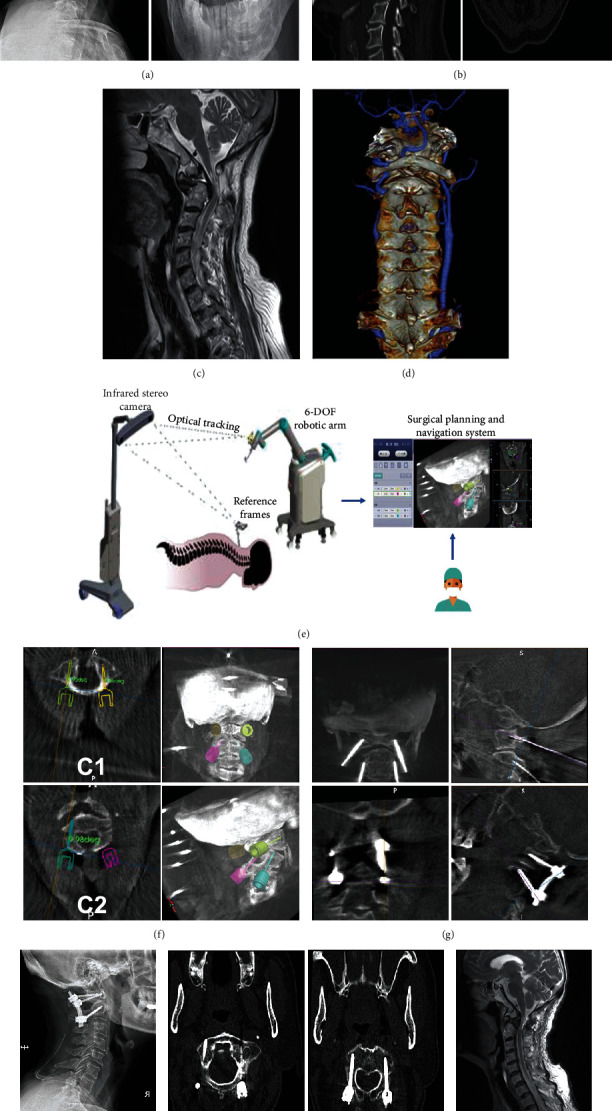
Case presentation. A 65-year-old female patient presented with a 3-year-long history of progressive dizzy, numbness, and weakness of extremities. (a, b) X-ray and CT examinations indicated os odontoideum and atlantoaxial instability (white arrows). (c) Preoperative magnetic resonance imaging (MRI) indicated a high signal intensity in the medulla oblongata. (d) No changes in VA course were observed in the 3D-CTA images. (e) The working principles of the TiRobot system. (f) Surgical planning of trajectories in the TiRobot system. (g) Robot-assisted insertion of C1LMS and C2PS. (h–j) X-ray, CT, and MRI images at 3 months postsurgery demonstrated that dislocation was repaired, compression was released, and there were no perforations of the screws.
